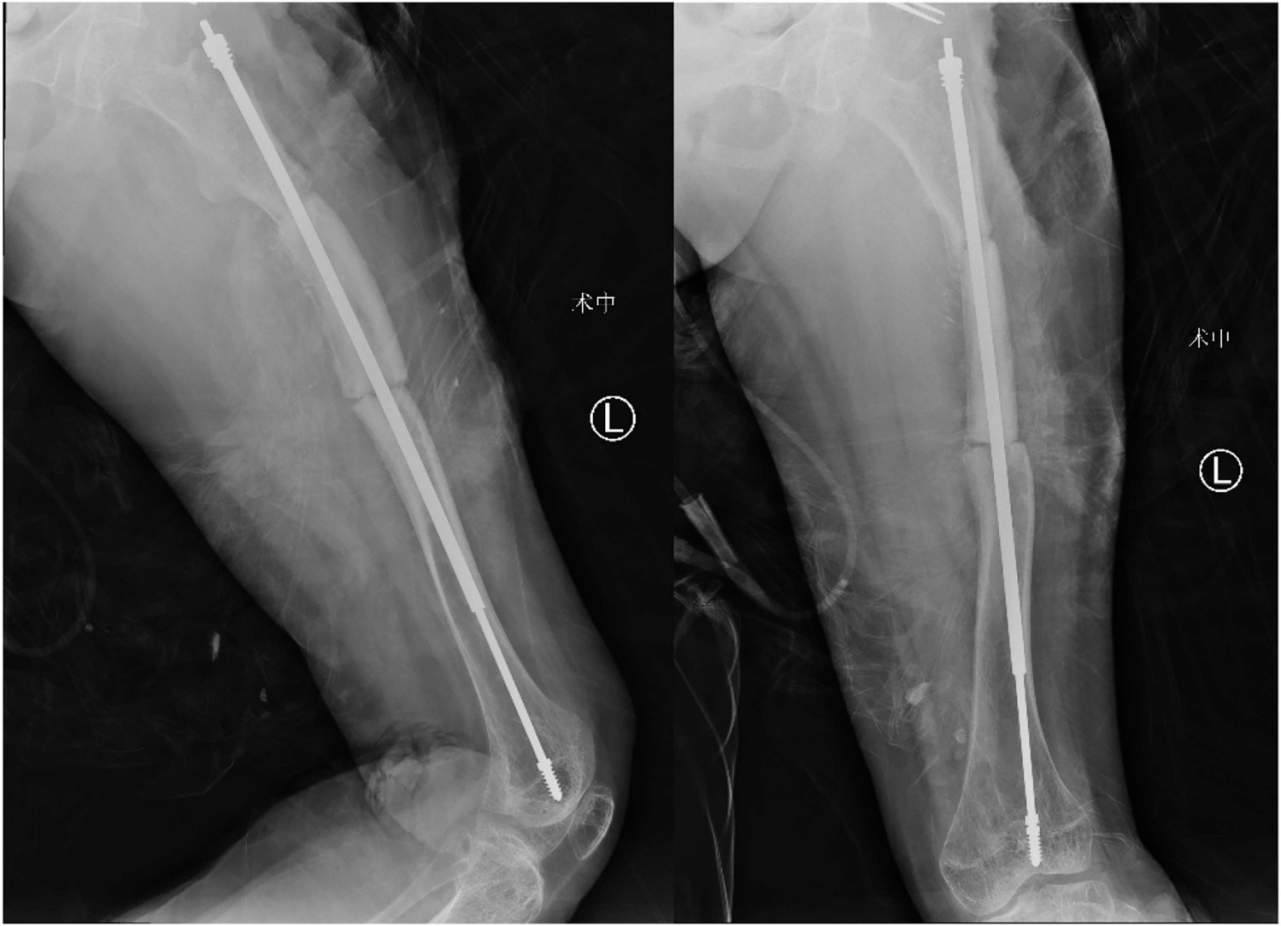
Postoperative review of the X-rays indicating that the left femur deformity had been corrected significantly, and the left lower limb force line recovered well.
